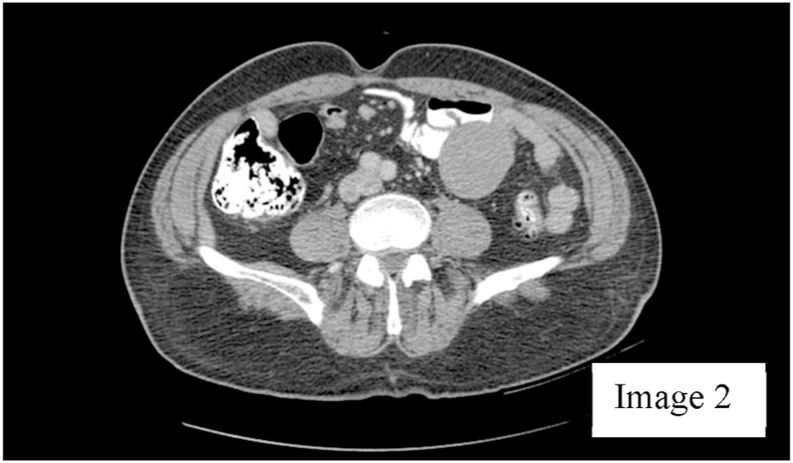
Axial plane of CT scan of abdomen.
